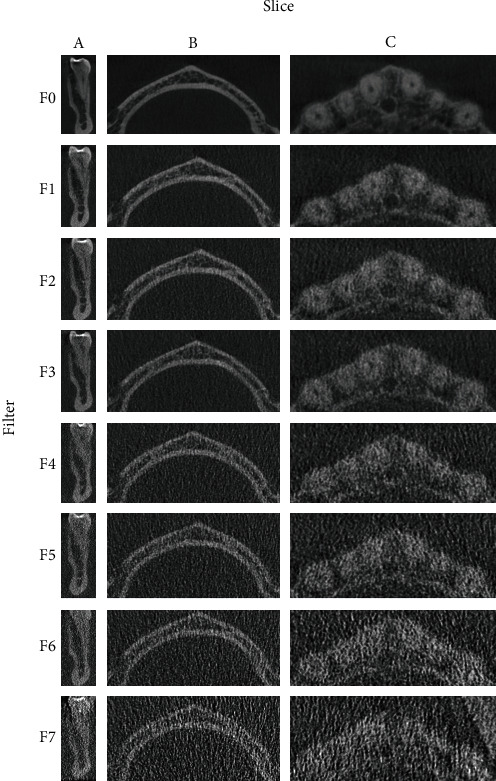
Overview of the 3 slices from 3D CBCT using filters F0-F7. Slice A, coronal view of the lower first molar; slice B, mandibular axial; slice C, maxillary axial.
